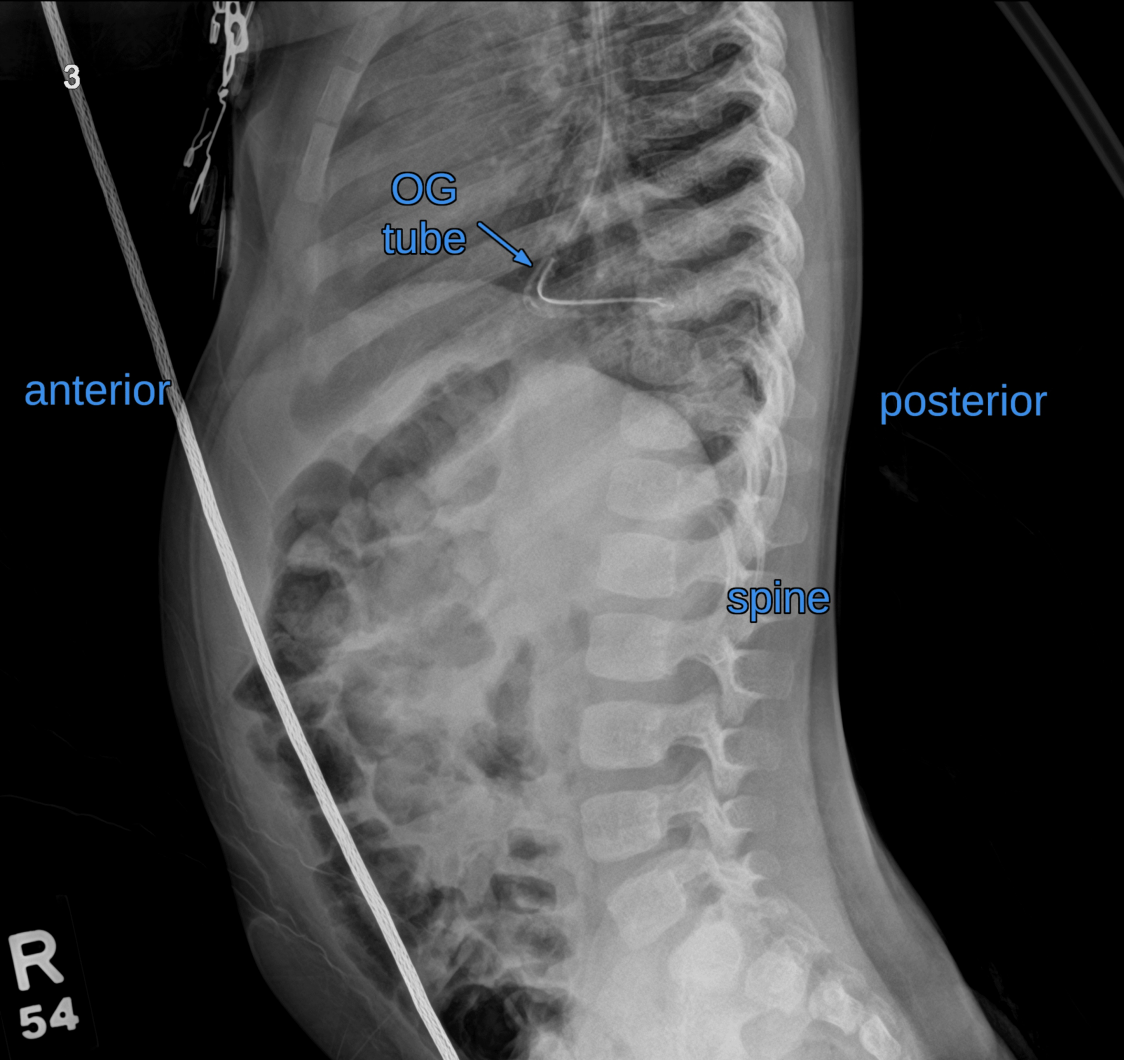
X-ray (lateral view) showing the orogastric (OG) tube with its tip towards the spinal column.
